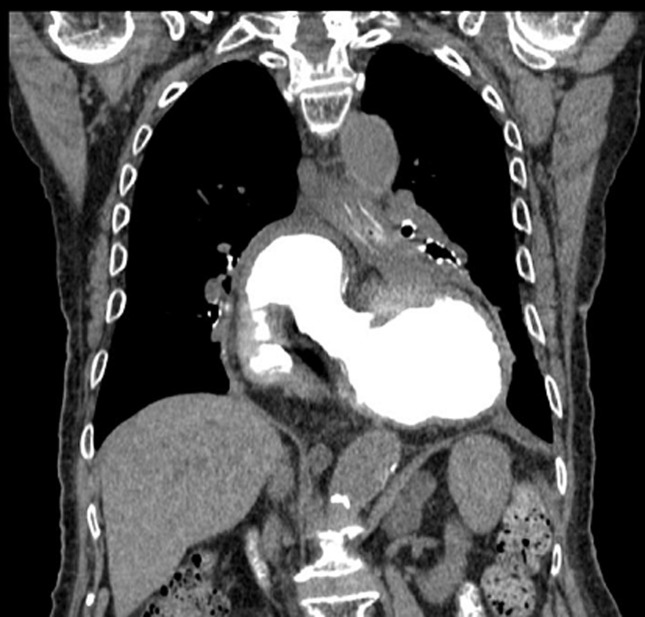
computed tomography (CT) scan showed the displacement of the stomach above the gastro esophageal junction; the stomach appeared upside-down with the antrum showing huge gastric distension with no free fluid or air
